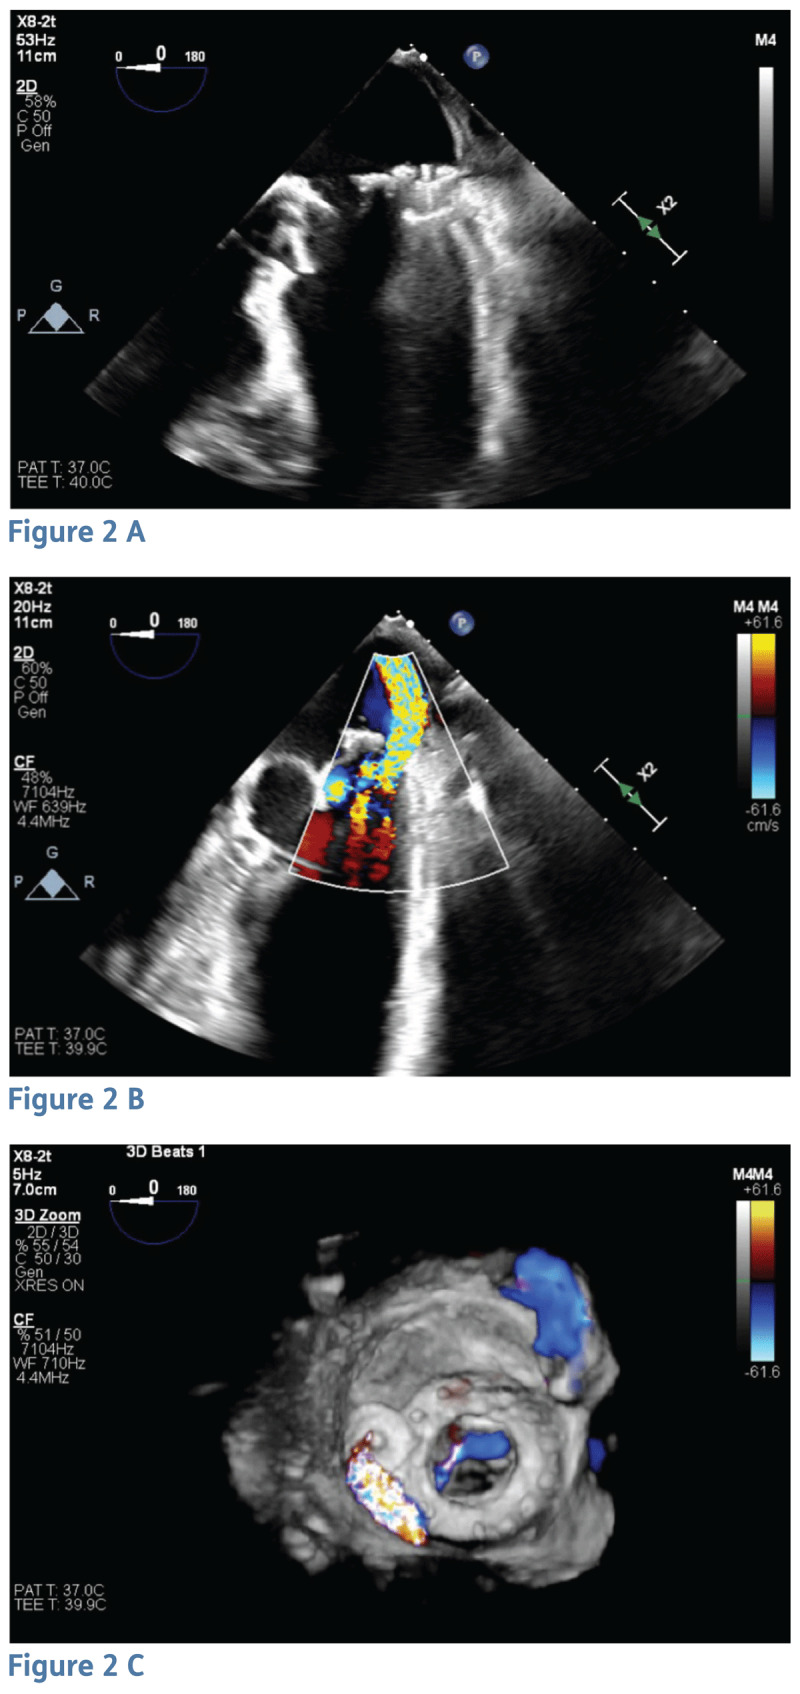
Transesophageal echocardiogram showing (A) septal occluder device in the lateral aspect of the bioprosthetic mitral valve, (B) color Doppler of modestly reduced paravalvular leak (PVL) after deploying septal occlude device, and (C) 3-dimensional image of reduced PVL.
